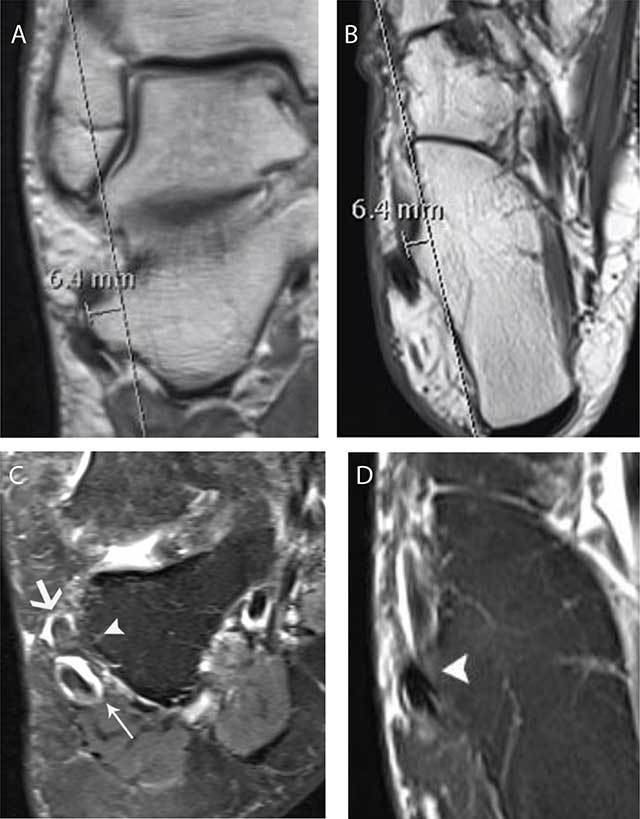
Hypertrophic TP and grade 1 tenosynovitis of the peroneus longus and brevis in a 64-year-old woman. Coronal PD (A), axial T1-WI (B), coronal FS T2-WI (C) and axial FS T2-WI (D) MR images. The hypertrophic tuberculum peroneum is measured on the coronal (a) and axial (b) PD images and has a width of 6.4 mm. The size is best measured on non-FS images as the cortical delineation is more precise than on the FS T2-WI. There is a focal thickening of the peroneus longus tendon (thin white arrow) with increased signal and effusion on the coronal (c) FS T2-WI MR images. Note also increased fluid within the tendon sheath of the peroneus brevis (thick white arrow) in keeping with mechanical friction. There is subtle bone marrow edema at the TP (white arrowhead). The axial (d) FS T2-WI MR images reveal also subtle bone marrow edema (white arrowhead).
